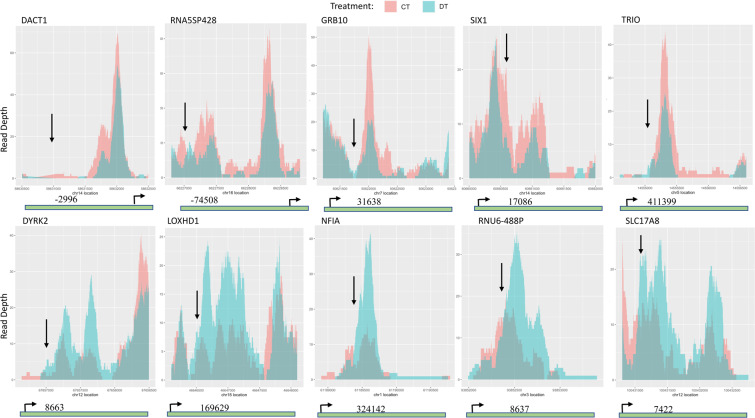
Top 5 genes modulated by DAC treatment. Overlay plots of control and DAC-treated cells showing the chromosomal locations of the peaks of acetylation decrease (upper panel) and acetylation increase (lower panel) of the top 5 genes. The gene map underneath each plot shows the distance from the start of the peak (indicated by the downward arrow) to the TSS (bent arrow). Gene maps are not drawn to scale. DT indicates DAC treatment and CT indicates control cells.
